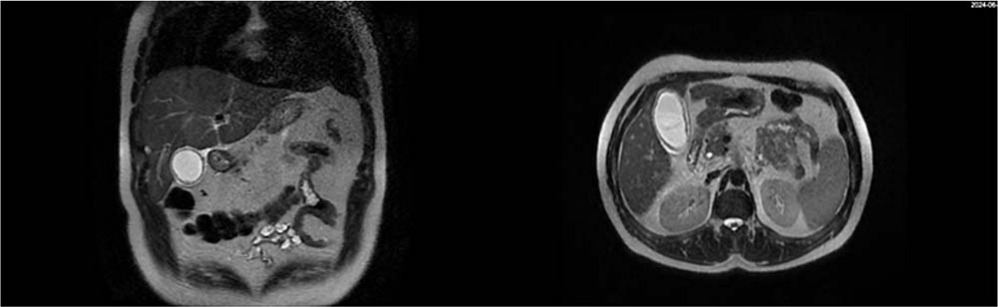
MRI showing wall thickening of the gallbladder, fluid around the gallbladder and a normal diameter of the CBD, as well as the rest of the biliary tree.
